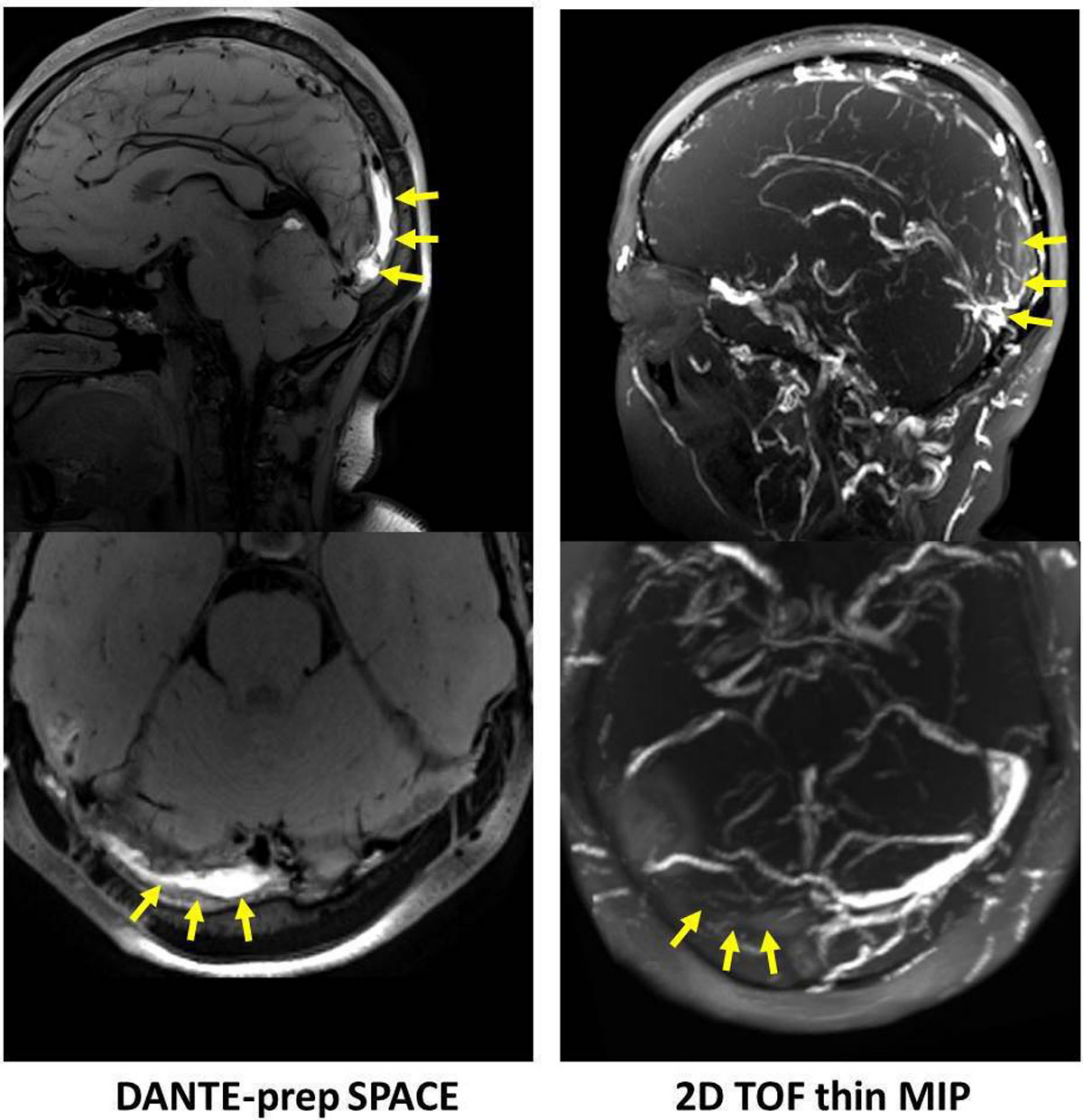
Patient study. Subacute CVT at the superior sagittal sinus and right transverse sinus is clearly depicted by DANTE-prepared T1-weighted SPACE. Note that hyper-intense signals on SPACE match flow voids on TOF as denoted by yellow arrows. The size of CVT is better appreciated on SPACE because of the positive contrast.
